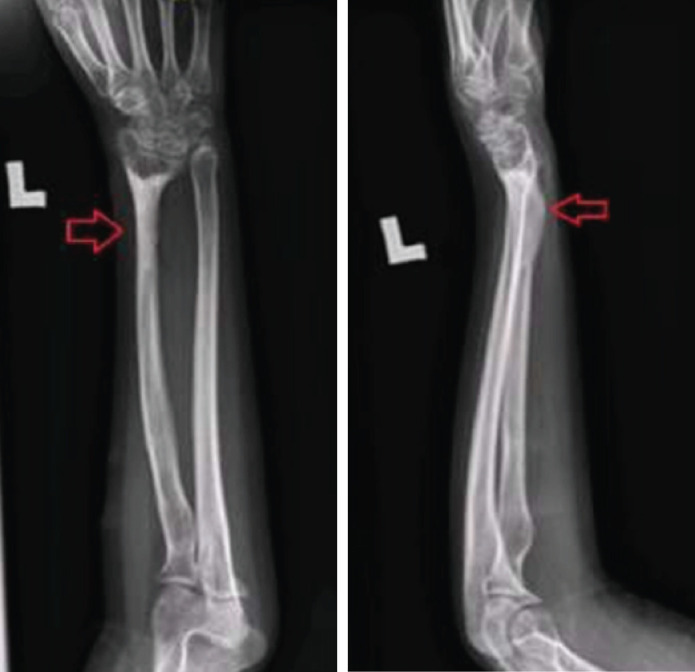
Radiograph after six months post-surgery right forearm after two months post-surgery.
